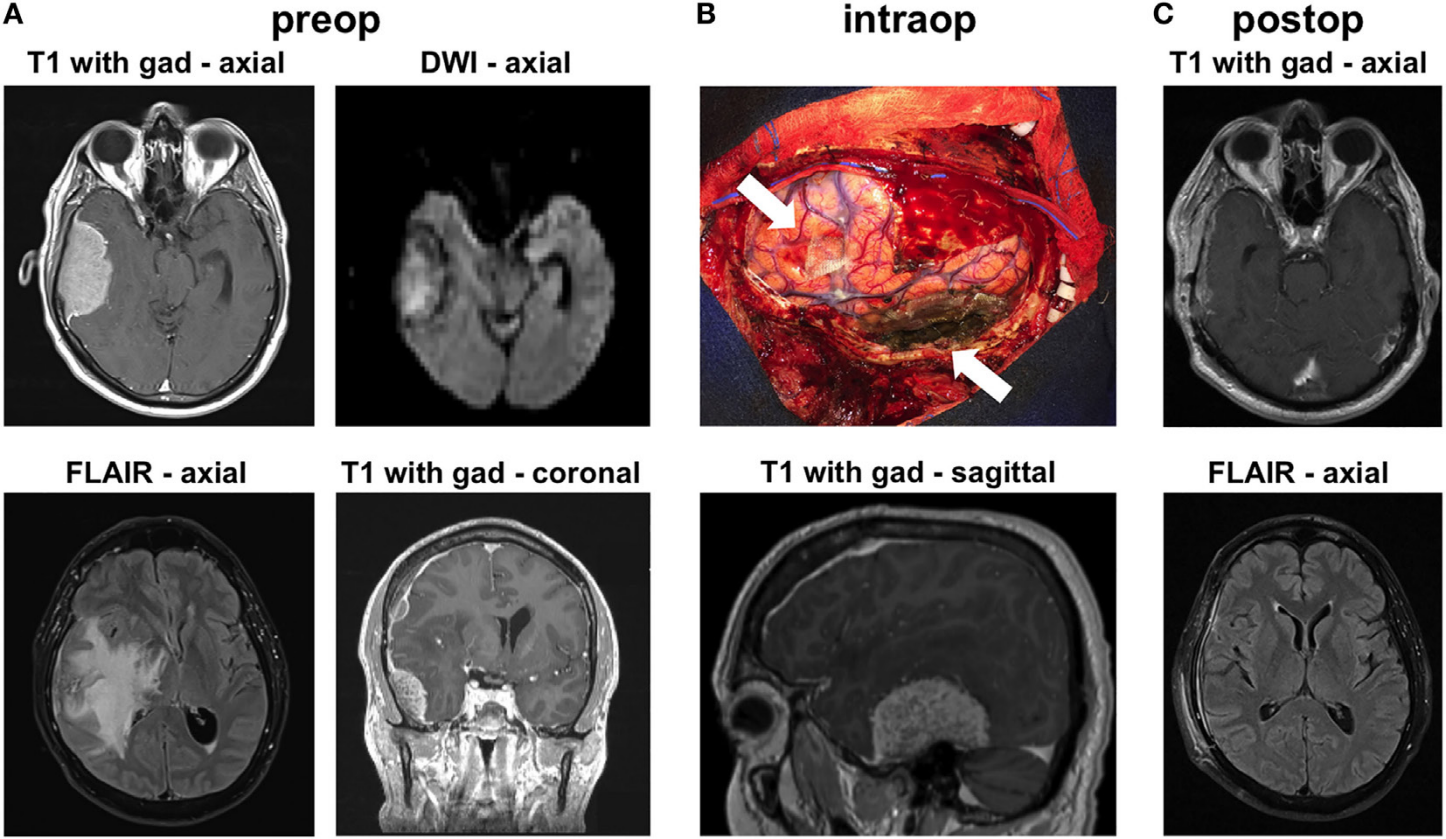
Radiographic findings. (A) Preoperative MRI shows contrast-enhancing extra-axial masses in the right temporal and frontal regions. The temporal mass shows diffusion restriction on diffusion-weighted imaging (DWI) imaging. There was substantial vasogenic edema within a large territory of the right hemisphere on FLAIR images. (B) Intraoperative image shows the areas of the two masses (arrows), after their resection. The image is shown in conjunction with a preoperative sagittal MRI image that highlights the location of the large temporal mass. (C) MRI 6 weeks after surgery shows no recurrence and resolution of the vasogenic edema on FLAIR imaging.
